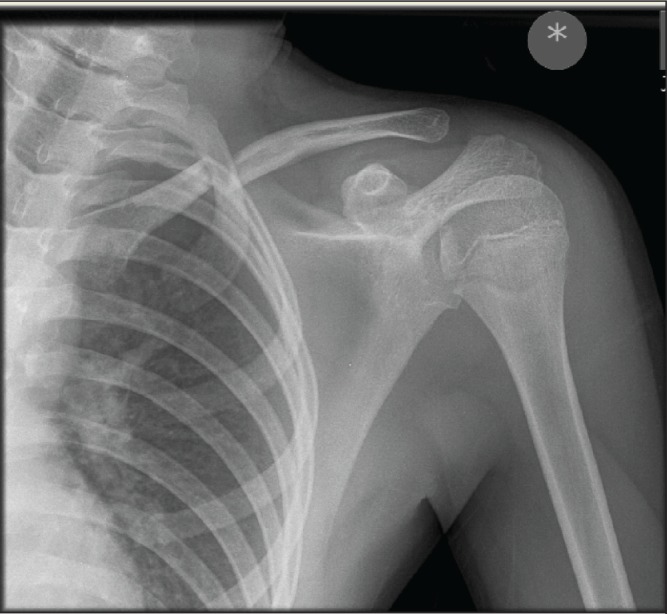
Plain x-ray showing midshaft clavicle fracture
